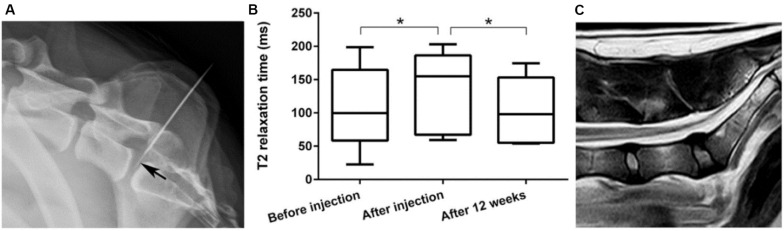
(A) Laterolateral radiographic images of fluoroscopy-guided intradiscal injection. Note that the 20-G needle advanced into the epidural space and the 27-G needle (arrow) positioned in the center of the NP. (B) T2 mapping values of the NP were significantly higher after injection compared with those at preinjection and 3 months’ follow-up. (C) Representative T2 magnetic resonance image 3 months after intradiscal injection with the celecoxib-loaded hydrogel. Reproduced from Tellegen et al. (2018) with permission from Copyright 2018 Wiley. p* < 0.05.
